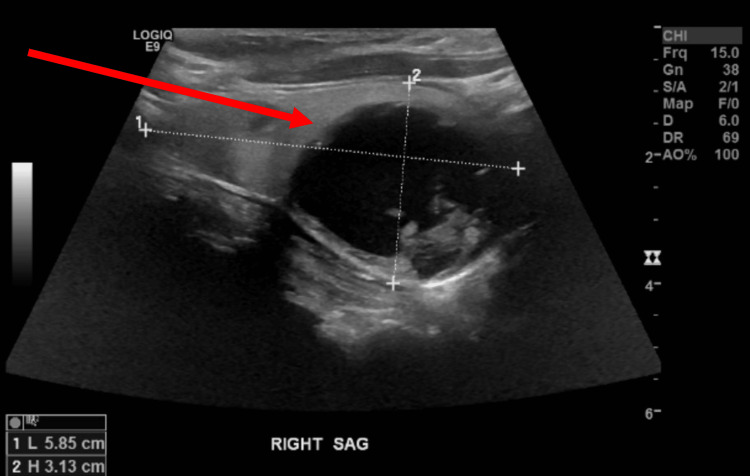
Thyroid ultrasound notable for a large dominant complex solid and cystic mass in the right thyroid gland (red arrow)
